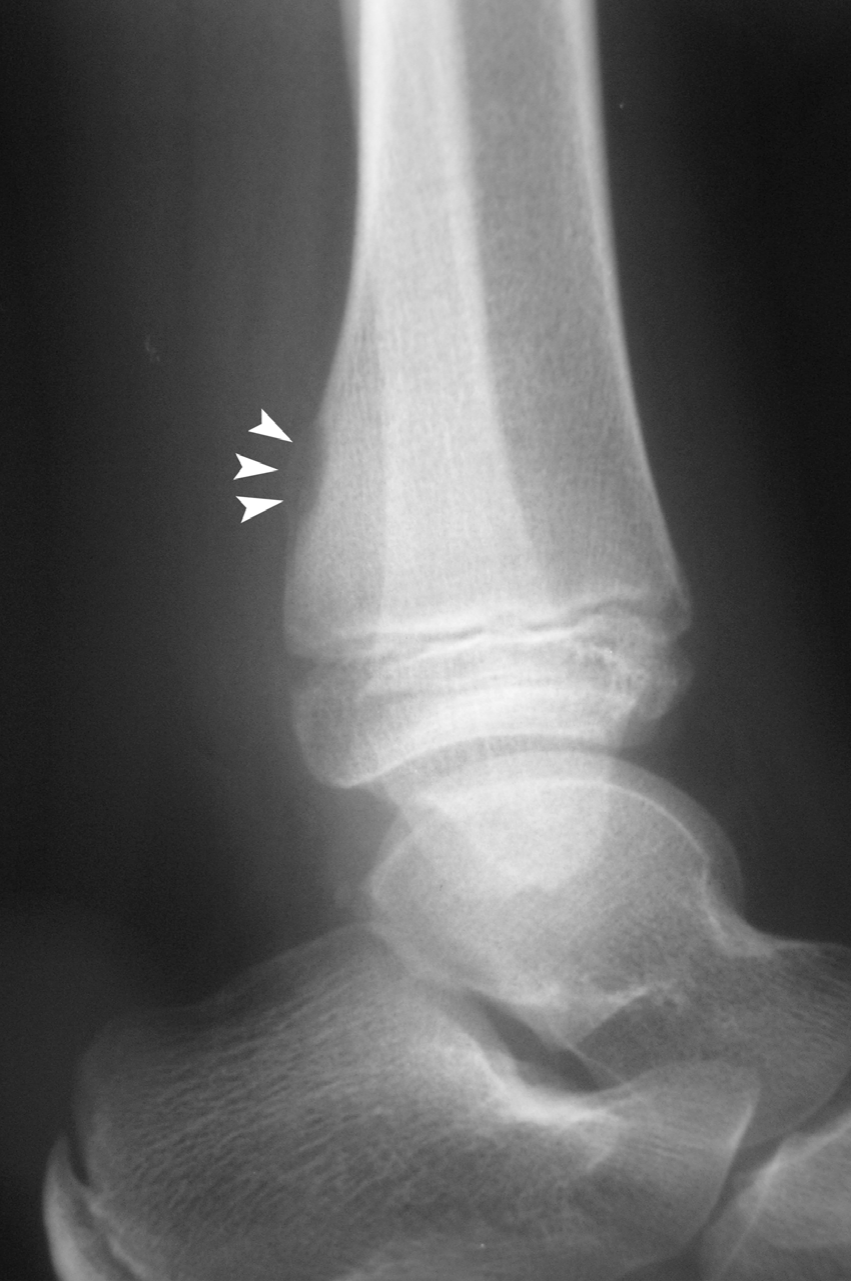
X-ray of the left ankle showing periosteal lesion with thin outer shell (white arrow heads).
